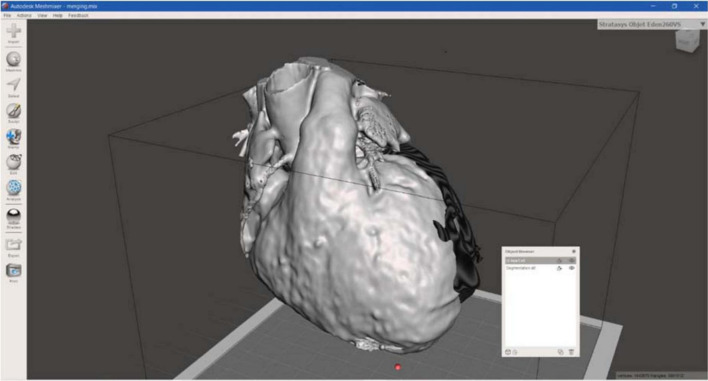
3D models merging. CT and CMRI models merging on the Autodesk Meshmixer software.
